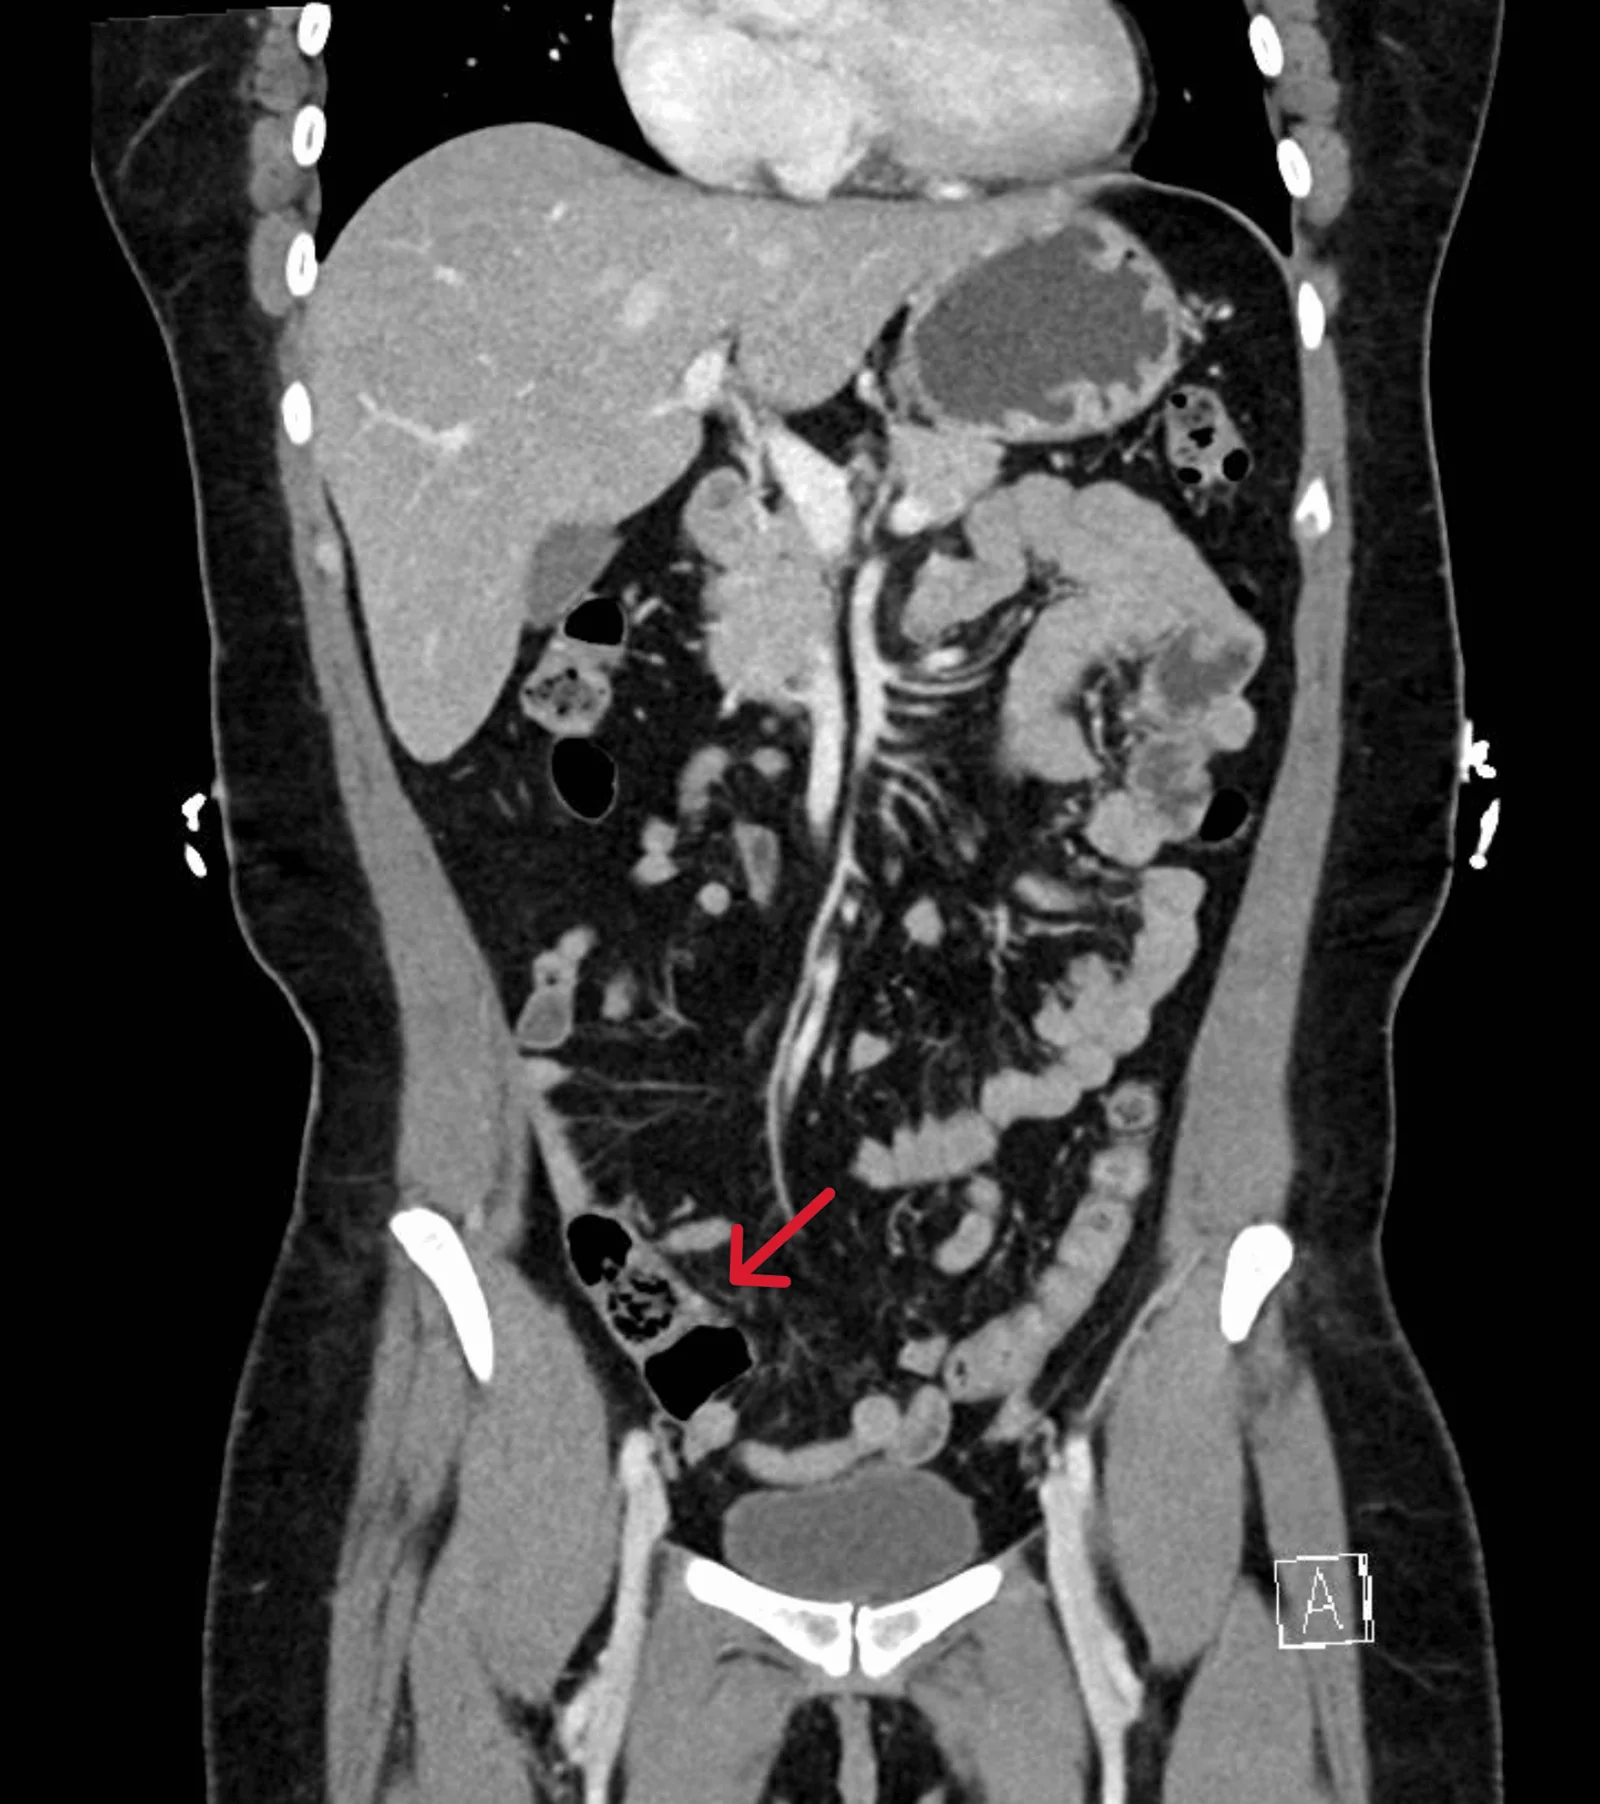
CT image of the abdomen and pelvis The CT examination demonstrated no inflammatory changes, free fluid, bowel distention, or bowel obstruction. The appendix was normal (red arrow). These findings supported the absence of an acute intra-abdominal process.
